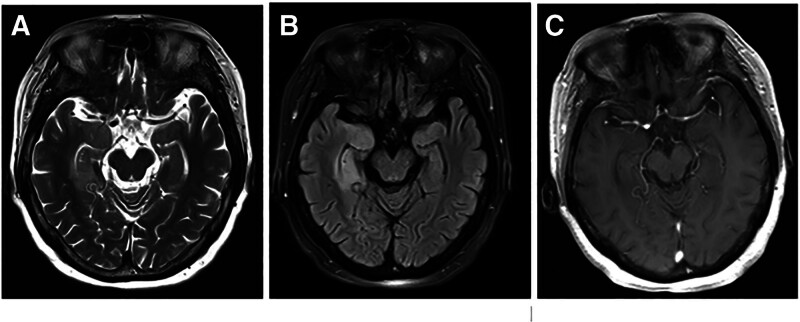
Repeat cranial MRI scan features of the patient after 3 weeks of efgartigimod treatment. (A) The abnormal signals in the medial aspect of the right temporal-occipital lobe on T2 -weighted sequence were attenuated compared to the baseline cranial MRI scan. (B) The abnormal signals in the medial aspect of the right temporal-occipital lobe on FLAIR-weighted sequence were attenuated compared to the baseline cranial MRI scan. (C) The lesions in the medial aspect of the right temporal-occipital lobe exhibit no contrast enhancement.
